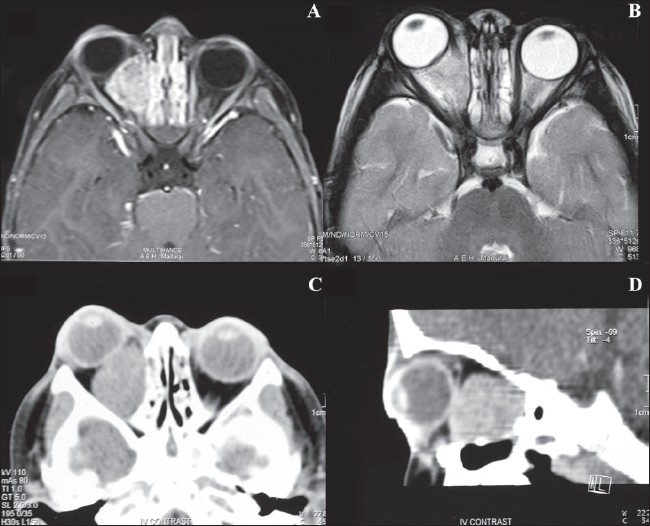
(A and B) MRI (T1 and T2 Wt Scan) suggestive of ovoidshaped mass which is hypointense on T1W and hyperintense in T2W/FLAIR sequences involving inferomedial quadrant of the right orbit. (C and D) CT scan shows increase in size of lesion involving extra and intraconal compartments of the right orbit engulfing the optic nerve sheath complex, medial rectus, inferior rectus and inferior oblique muscles
